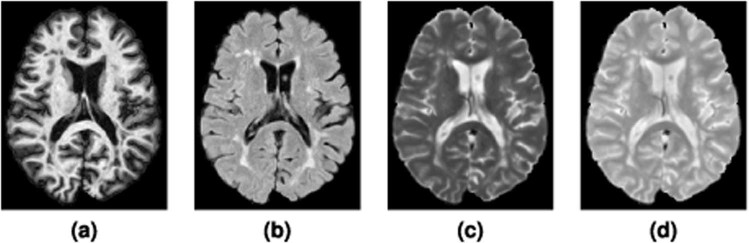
Shown are the (a) MPRAGE, (b) FLAIR, (c)
